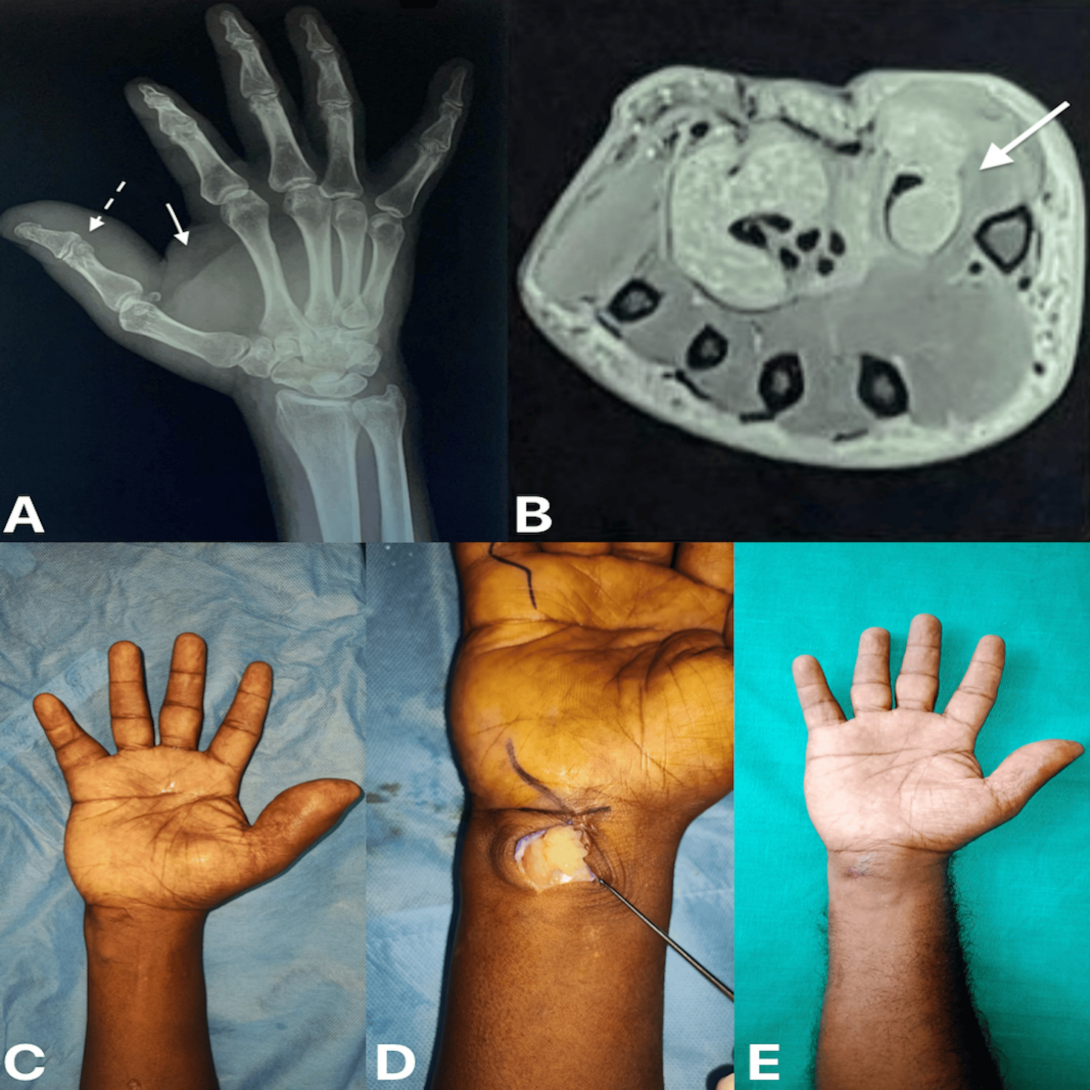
(A) Radiograph of the right hand with wrist: oblique view, (B) magnetic resonance imaging of right wrist: axial section, (C) pre-operative clinical picture of the right hand with wrist, (D) intra-operative picture showing rice bodies, and (E) one-year post-operative clinical picture of the right hand with wrist (A) The dotted arrow shows thumb interphalangeal joint destruction and erosion. The bold arrow shows a soft tissue shadow over the thumb and thenar region. (B) Arrow denotes synovial thickening in the palmar bursa.
